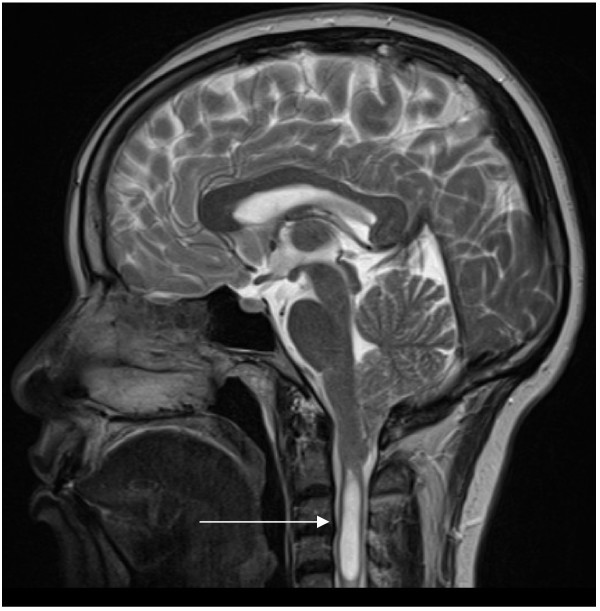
Patient 11. T2 weighted saggital MRI brain and cervical cord showing marked Chiari 1 malformation and large cervical syrinx.*
